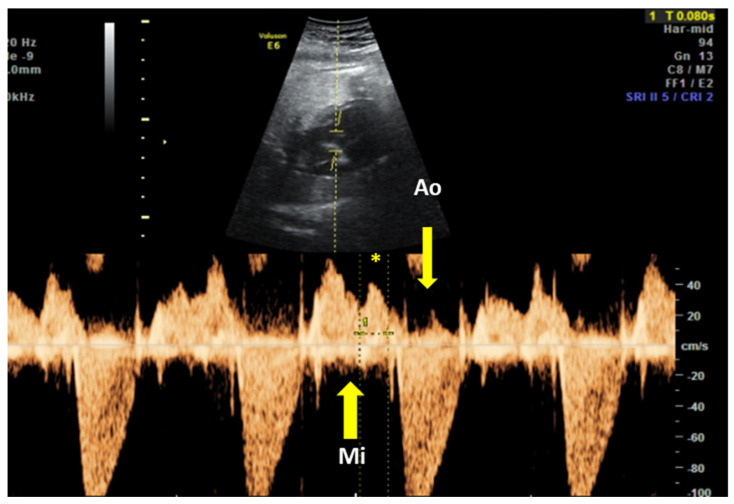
Pulsed wave Doppler trace used for mechanical PR interval measurement. Mi—transmitral flow (thick yellow arrow); Ao—transaortic flow (thin yellow arrow); (*)—normal PR interval—120 msec (between thin dotted yellow lines). Courtesy of Anca Panaitescu, Filantropia Clinical Hospital, Bucharest.
